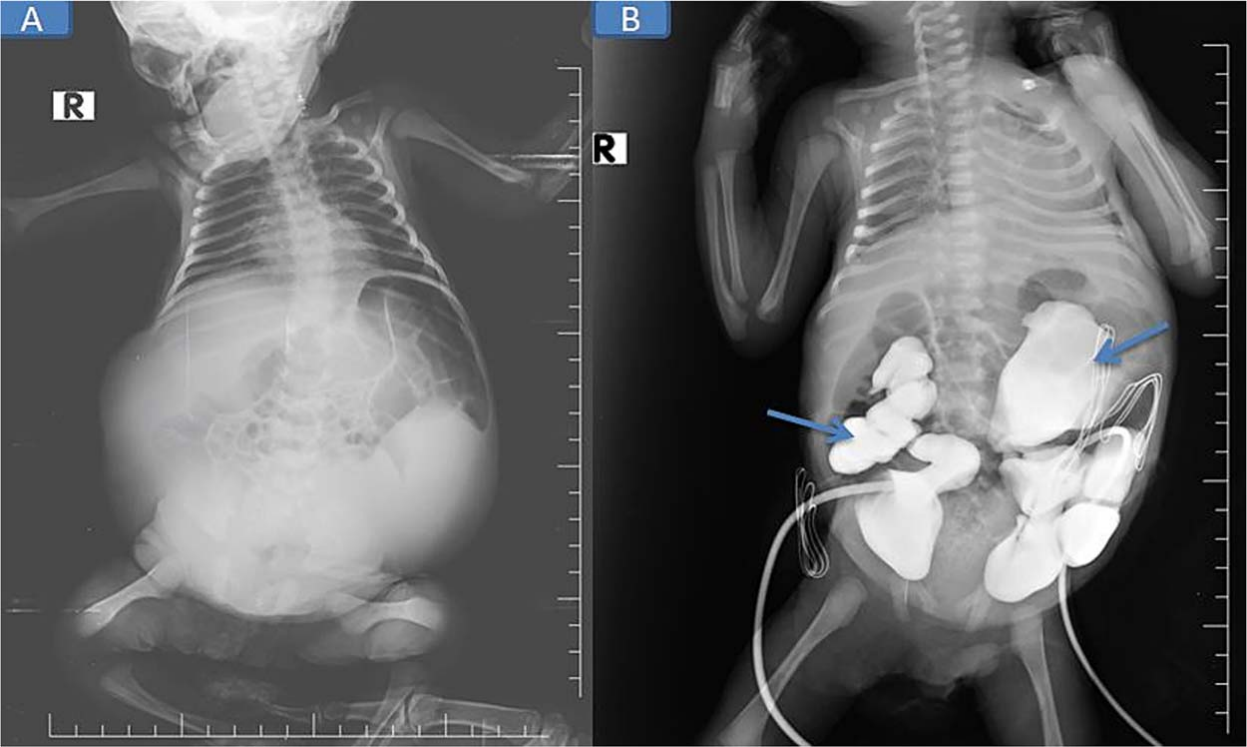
Pre-op abdominal X-ray (A) and post-operative percutaneous nephrostomy procedure with contrast injection through the nephrostomy (B).
